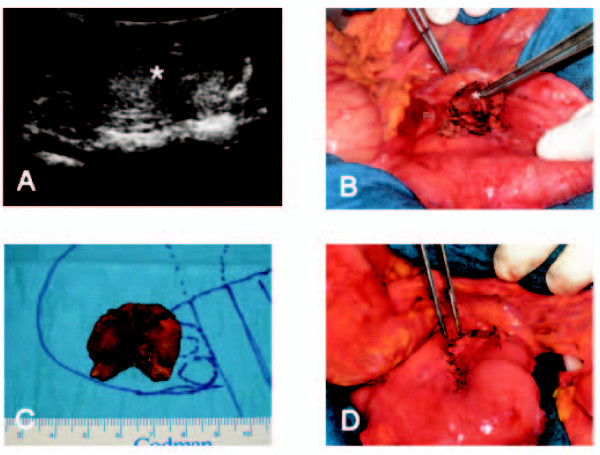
Situs at laparotomy before and after resection. (A) Intra-operative ultrasound at laparotomy. (B) Situs at laparotomy (asterisk indicates the tumor; S, stomach; P, pylorus; PH, pancreatic head; D, duodenum). (C)Macroscopic view of the resected tumor. (D) Covering of the defect after resection by a Y-en-Roux jejunal loop.
